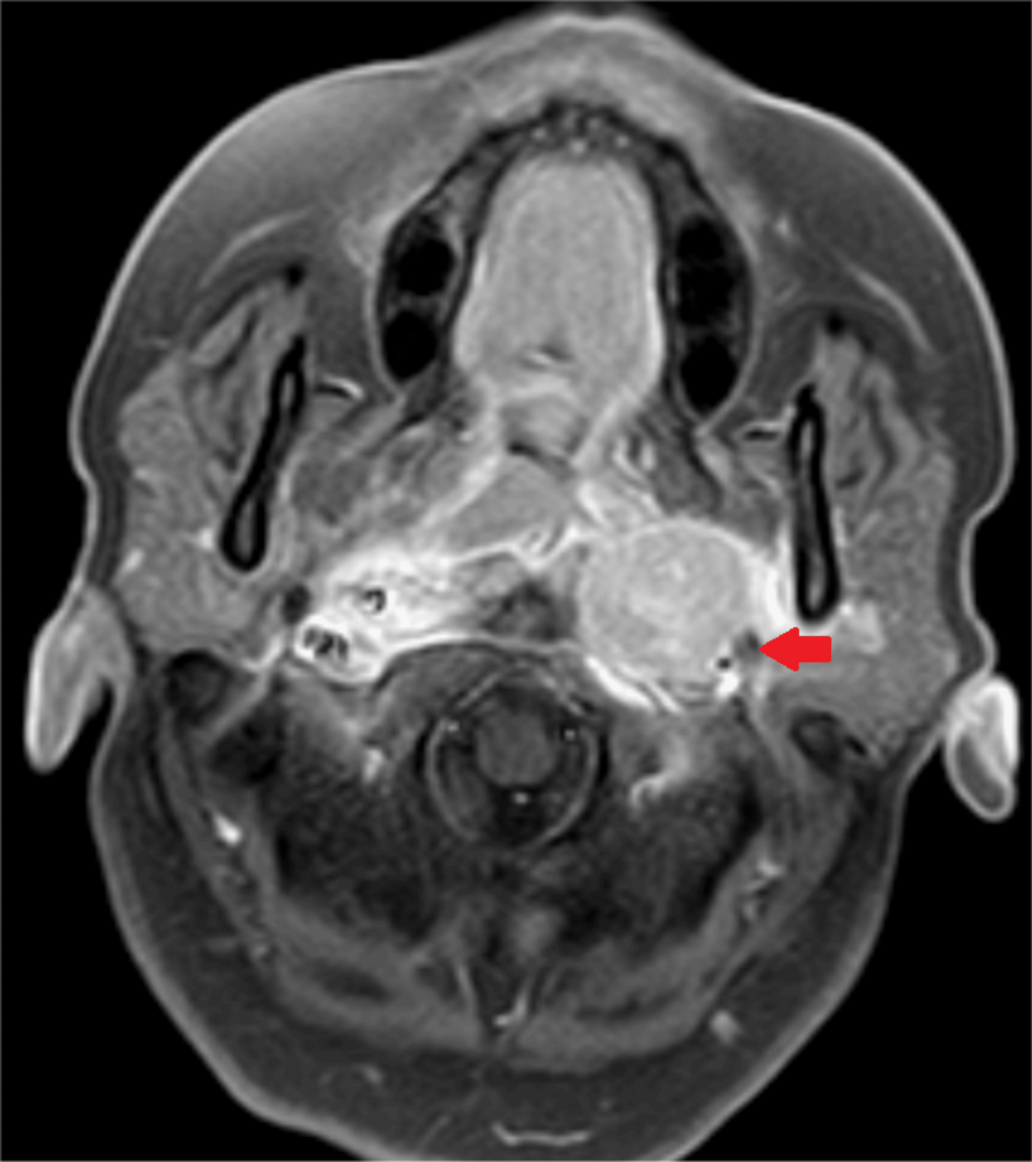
Contrast-enhanced MRI brain with inner ear Case 1: This axial section shows a hyperintense mass lesion in the nasopharynx (red arrow) that extends along the posterior nasopharyngeal wall and involves pharyngeal mucosal space with adjacent similar homogenous enhancing nodal masses in bilateral parapharyngeal space. MRI, magnetic resonance imaging
